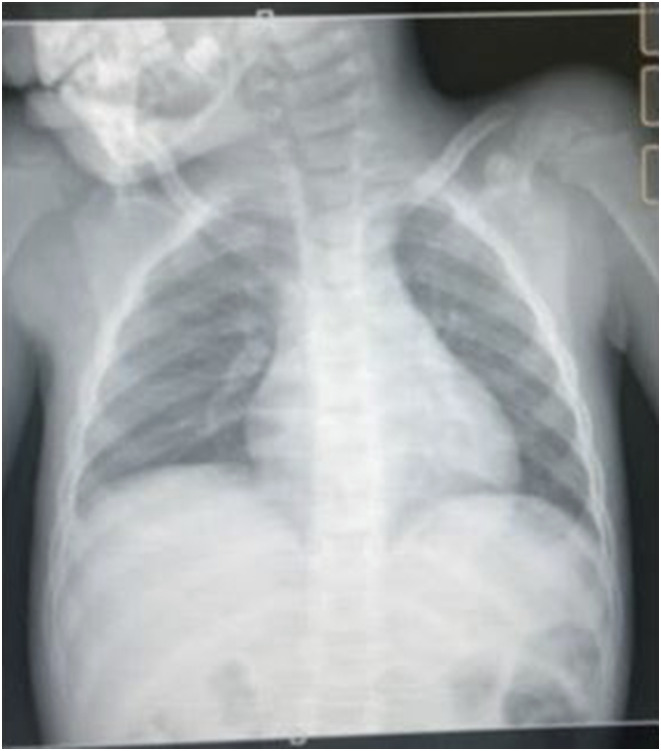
Chest X-ray of patient 4.
